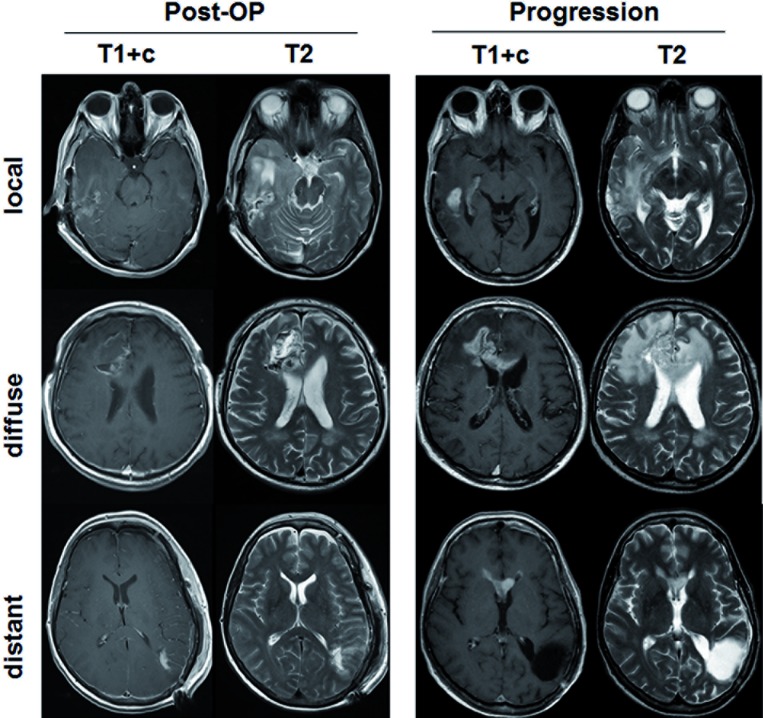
Represantative images for patterns of progression MRI scans for the three types of progression (local, diffuse, distant), as predefined in Methods, are shown. The first two columns show the postoperative situation on contrast enhanced T1 sequences (T1+c) and T2 weighted sequences. The last two columns show the corresponding scans at the time of first progression.
